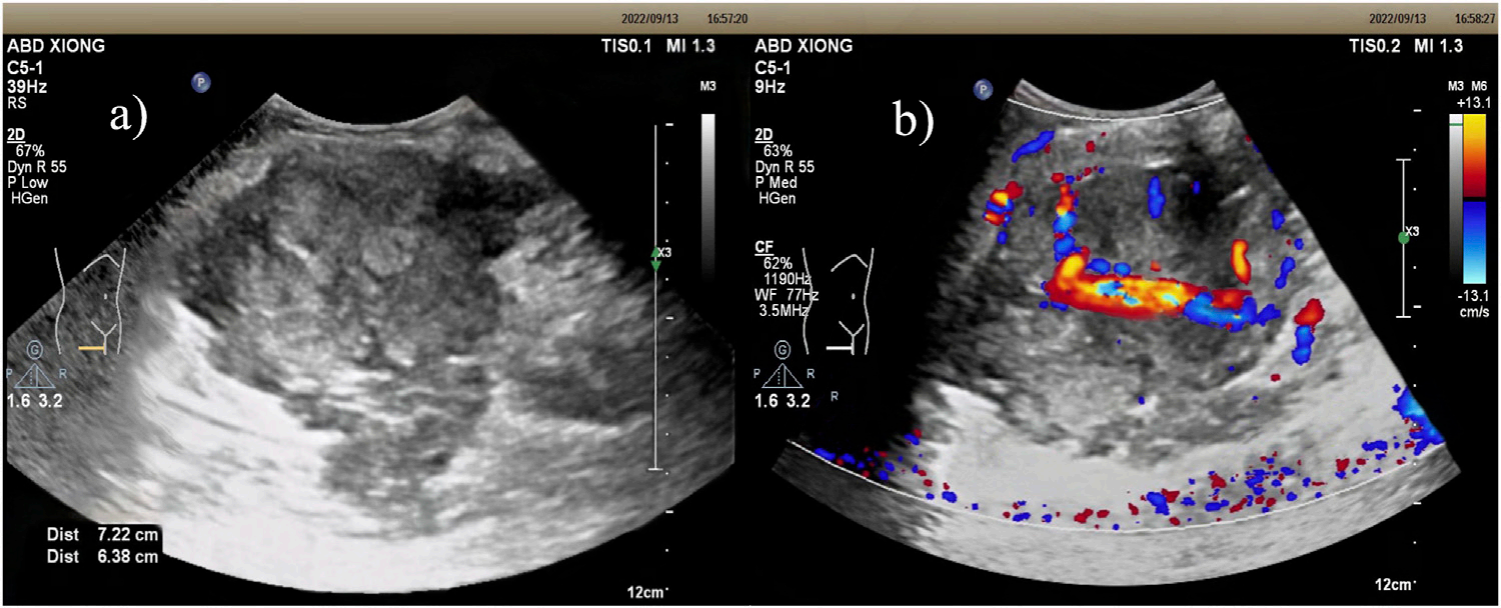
Ultrasound: (A) Slightly hypoechoic mass within the vastus medialis muscle of the right middle and lower thigh, with irregular morphology, poorly defined borders, and poorly homogeneous internal echogenicity. (B) Color Doppler: richer blood flow signals were seen within the mass.
